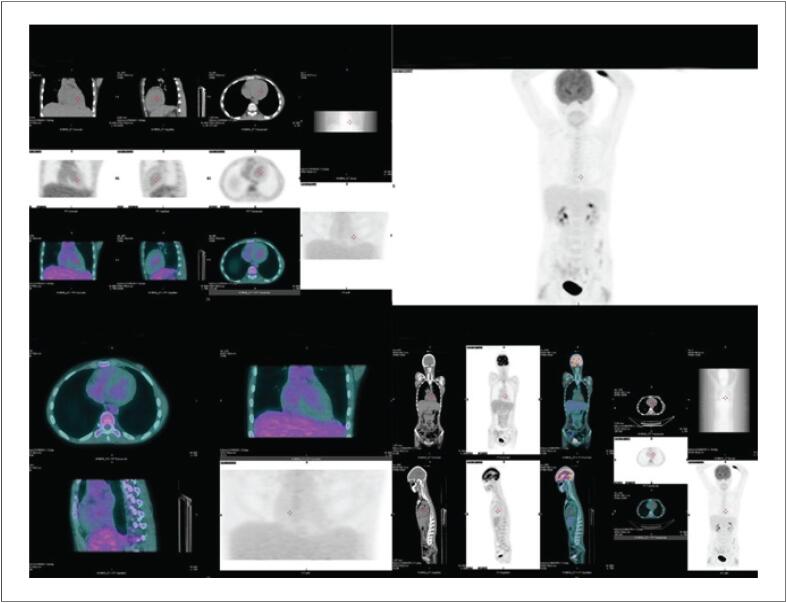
^18^F-FDG PET-CT na miocardite lúpica pós-tratamento: Observa-se regressão completa da captação após 2 meses de tratamento com imunossupressor.
